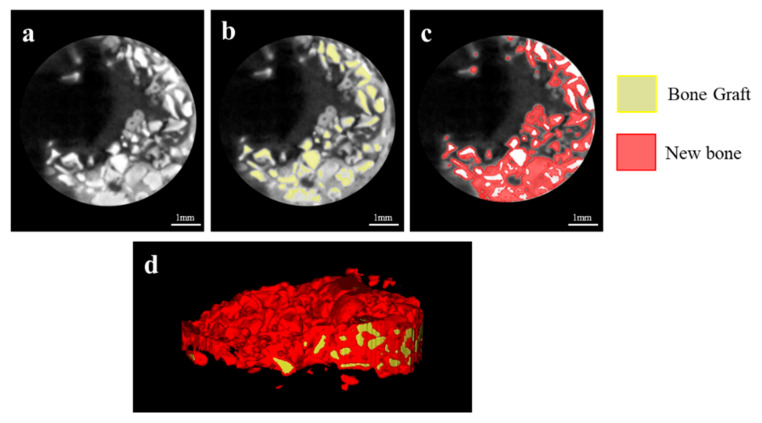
Images within ROIs obtained by micro-computed tomography analysis. (a) Two-dimensional micro CT image; colored images of (b) grafted bone scaffold and (c) new bones. (d) Converted 3D image for adjudging external appearance.
